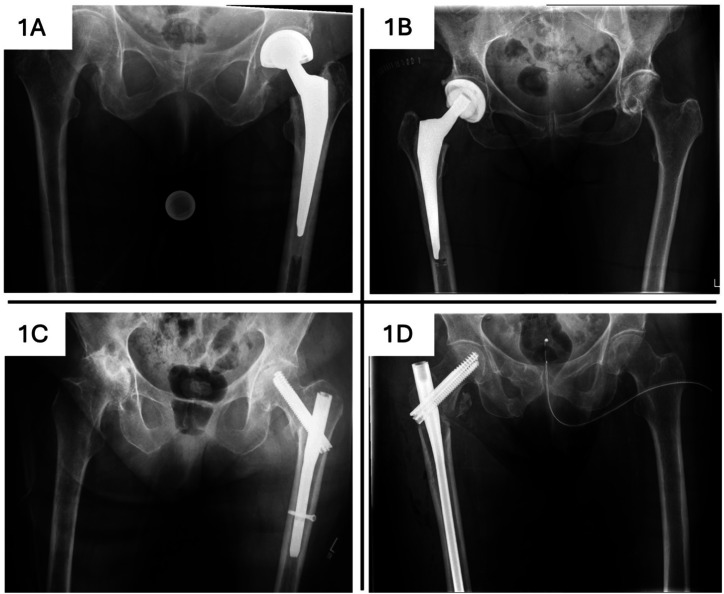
Representative radiographs of the implants used in this study. Cemented hemiarthroplasty of the left hip (a), Total hip arthroplasty in hybrid technique with a press-fit cup and a cemented stem (b), Short cephalomedullary nail (c), Long cephalomedullary nail (d).
